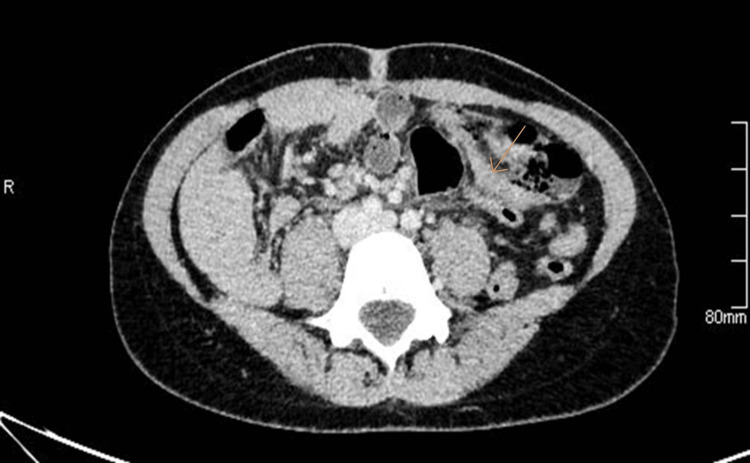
Axial CECT demonstrates a left-sided appendix arising from the malrotated cecum, measuring approximately 9 mm, with periappendiceal fat stranding, consistent with acute appendicitis. The arrow indicates the appendix arising from the malrotated cecum. CEDCT: contrast-enhanced computed tomography.
